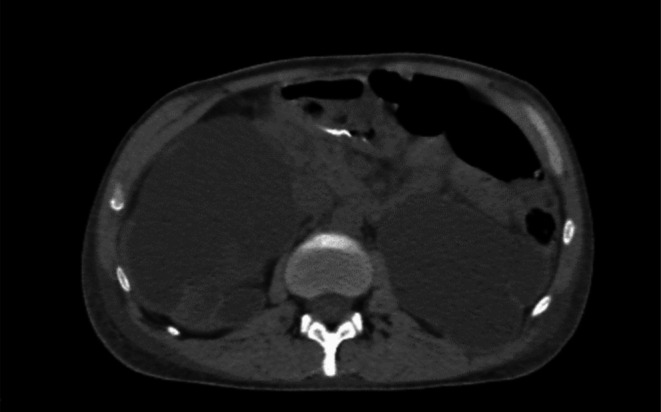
Abdominopelvic CT scan showing severe bilateral hydronephrosis.
